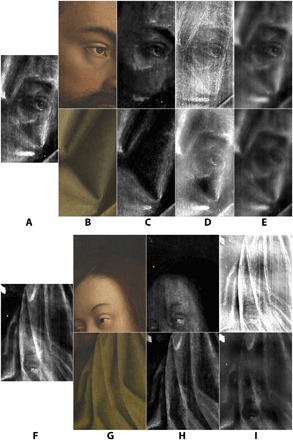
Comparison between the results generated with the proposed new algorithm and the preceding state-of-the-art results for a detail of the *Adam* panel. Top: (A) the mixed x-ray; (B) the RGB images from each side of the panel (before conservation) corresponding to the x-ray detail (i.e., the algorithm inputs); (C) reconstructed x-ray images produced by the proposed algorithm; (D) reconstructed x-ray images produced in (*25*); and (E) reconstructed x-ray images produced by coupled dictionary learning (*30*). All of the grayscale images presented here have gone through histogram stretching to have a common ground for the comparison. *Eve* panel (bottom): (F) the mixed x-ray; (G) the RGB images from each side of the panel (before conservation) corresponding to the x-ray detail (i.e., the algorithm inputs); (H) reconstructed x-ray images produced by the proposed algorithm; and (I) reconstructed x-ray images produced in (*30*). All of the grayscale images presented here have gone through histogram stretching to have a common ground for the comparison.
